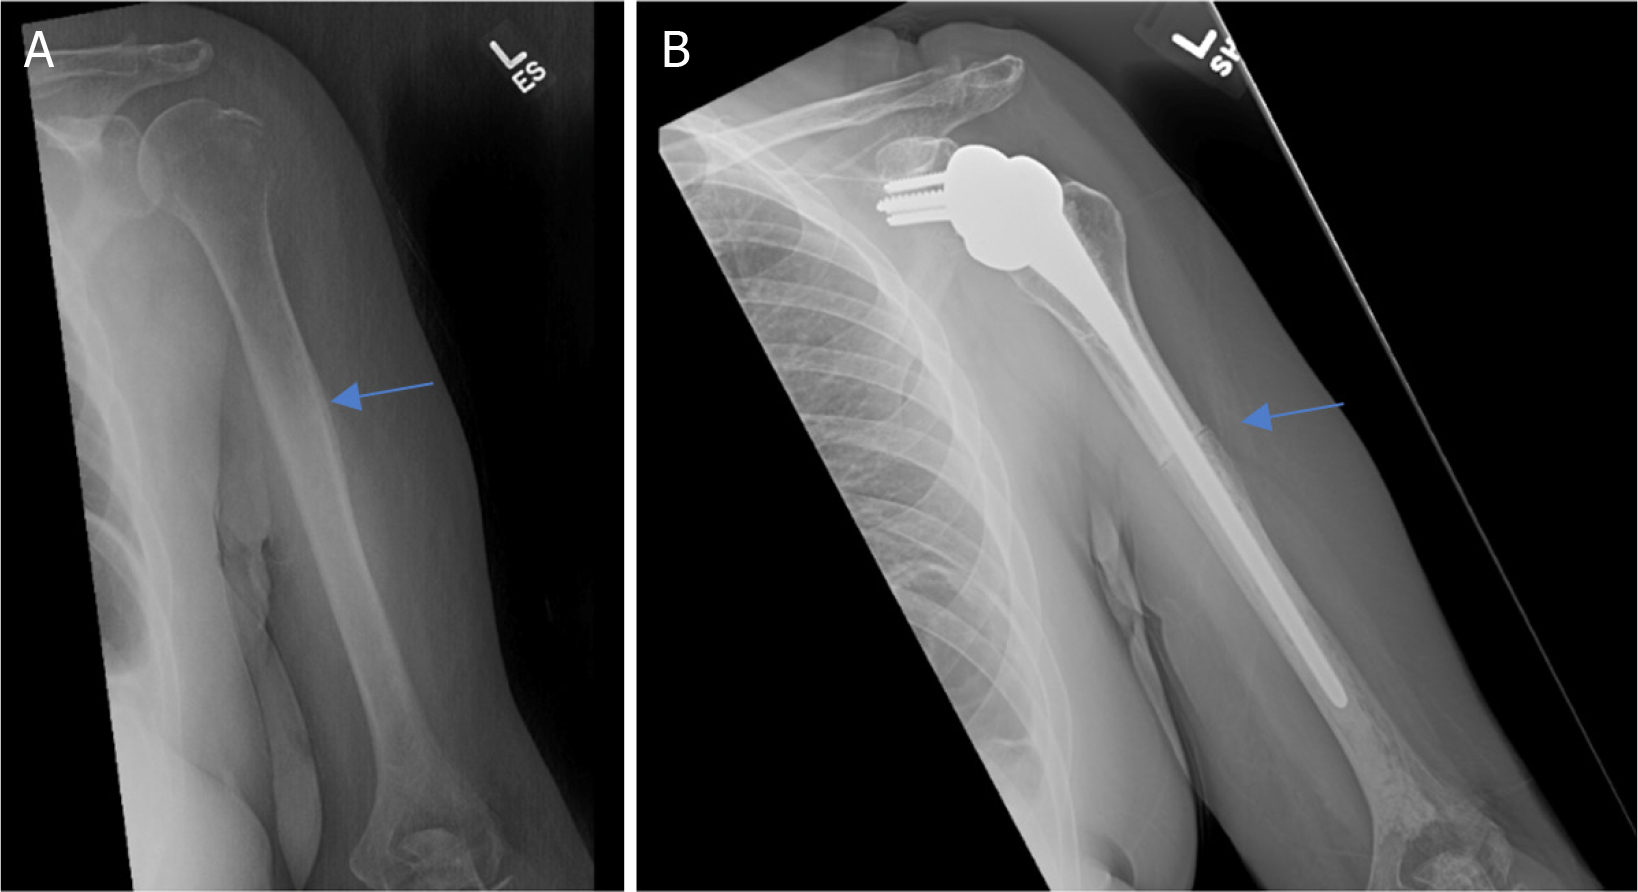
Anteroposterior radiographs of a 50-year-old female with: a solitary renal cell carcinoma tumor of the proximal humerus preoperatively (A); one year after wide excision and proximal humeral APC reconstruction (B). Blue arrow notes the junction between the Allograft and the native bone
